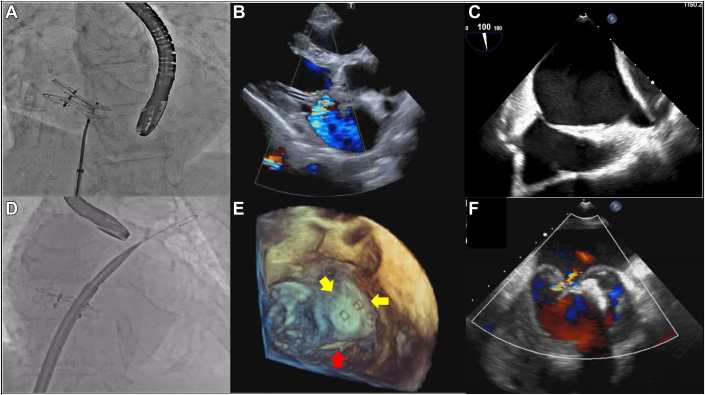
Key Imaging Findings in TEER After Prior Septal Closure (A) Fluoroscopic image from 1 year earlier showing transcatheter closure of the patent foramen ovale and atrial septal defect using 2 occluder devices. (B) Transthoracic echocardiography on day 1 demonstrating severe degenerative MR due to P1 prolapse with ruptured chordae. (C) TEE in the bicaval view at the time of TEER, demonstrating stretching of the interatrial septum. (D) Fluoroscopic image showing passage of the PASCAL Ace delivery system through the interatrial septum. (E) Three-dimensional TEE image showing the PASCAL Ace guiding sheath (red arrow) crossing the interatrial septum, with 2 previously implanted septal closure devices also visualized (yellow arrows). (F) TEE image showing mild residual mitral regurgitation after transcatheter edge-to-edge repair. MR = mitral regurgitation; TEE = transesophageal echocardiography; TEER = transcatheter edge-to-edge repair.
